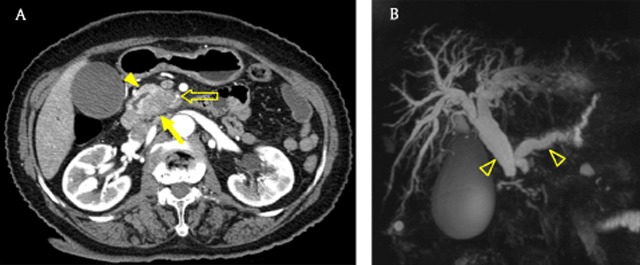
A 79-year-old woman with pancreatic ductal adenocarcinoma. (A) Axial pancreatic phase CT image shows lower attenuating mass (arrow) compared with the pancreas parenchyma (arrowhead) in the pancreatic head, which encases the first jejunal branch of the superior mesenteric artery (open arrow). (B) Magnetic resonance cholangiopancreatography (MRCP) image shows dilatation of the bile and pancreatic ducts, the so-called double duct sign (open arrowheads). Note the abrupt narrowing of both bile and pancreatic ducts.
